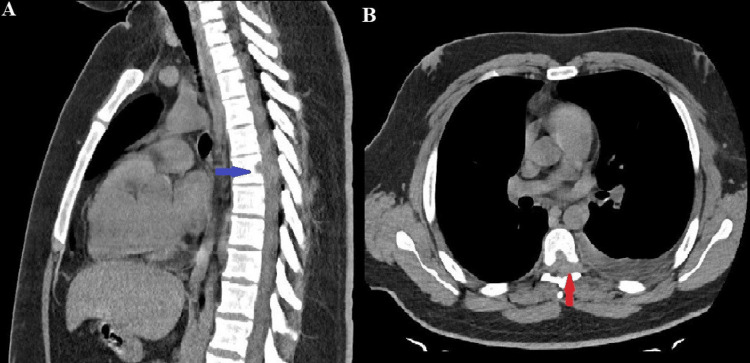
(A and B). A segment of a native CT scan of the thoracic spine in the sagittal and axial planes. Figure 2A shows the destruction of the body of TH7 vertebra and prolapse of the isodense formation towards the dural sac (blue arrow). Figure 2B presents an axial section through the level of the TH7 vertebra and the presence of an isodense formation filling the posterolateral (left) epidural space, with the border between the spinal cord and the epidural space obliterated (red arrow).
